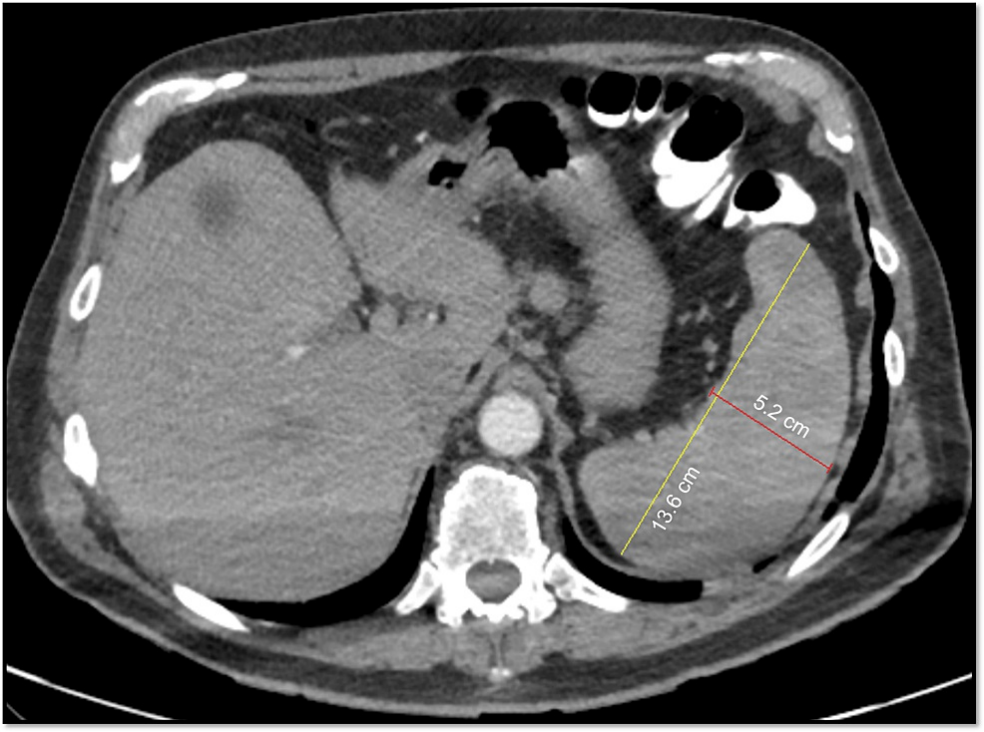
CT abdomen revealing splenomegaly (13.6 cm x 5.2 cm)
